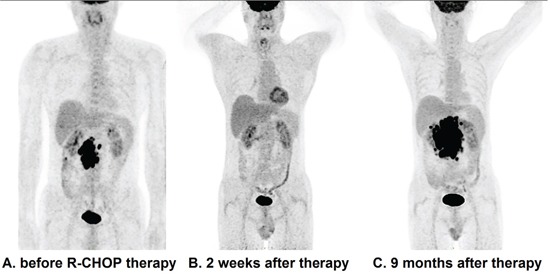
^18^F-FDG PET images of a recurrent case The baseline PET image shows high tumor burden with TLG of 1244g A. Although no hyper-metabolic lesions were seen on PET images after 6 cycles of R-CHOP therapy B., the patient experienced relapse nine months after the R-CHOP therapy C.
